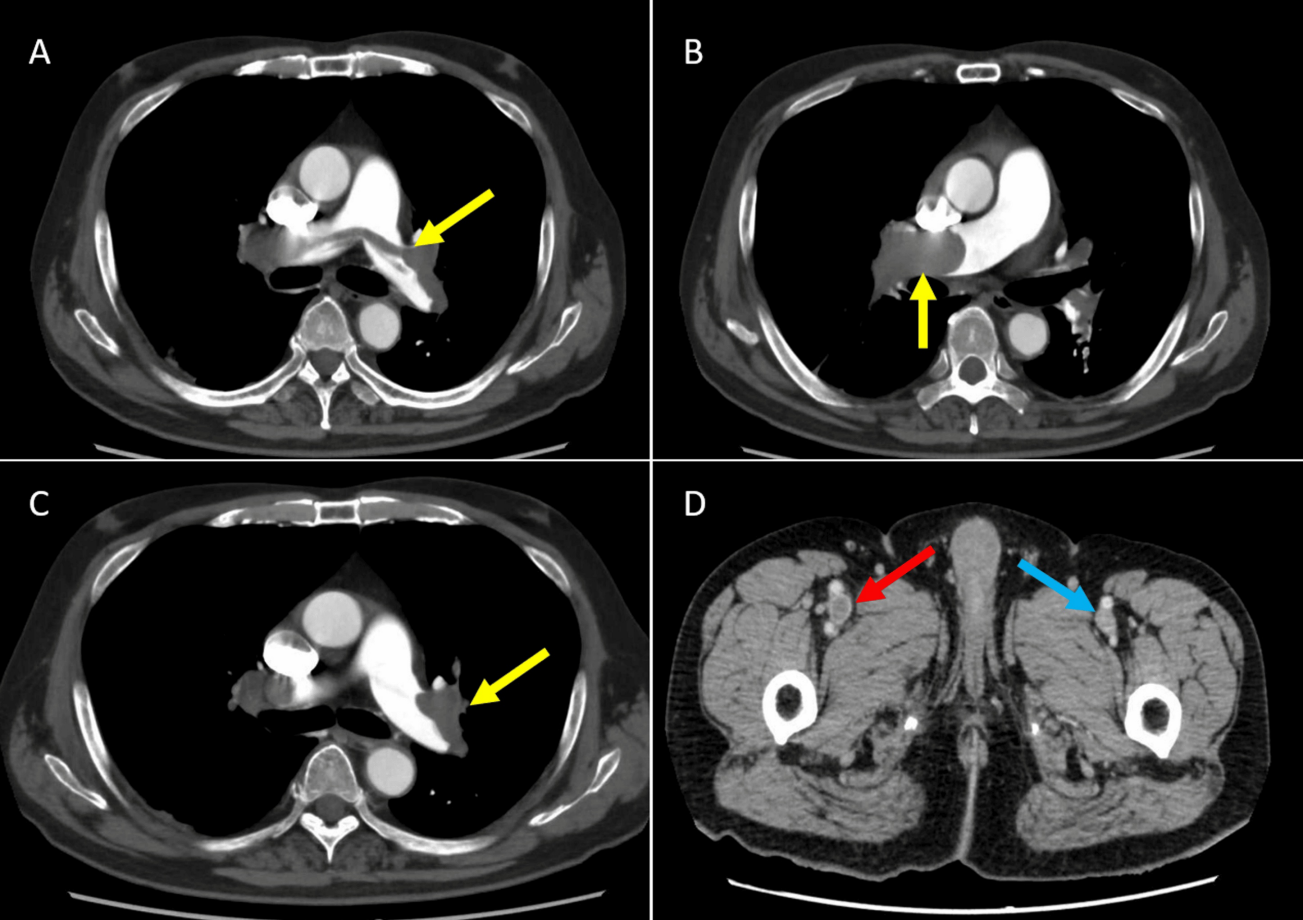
CT scan images at admission Contrast-enhanced CT images at presentation show evidence of acute pulmonary embolism and deep vein thrombosis. A, B, and C demonstrate filling defects within the pulmonary arteries (yellow arrows), consistent with bilateral thromboembolism. D shows a filling defect in the right femoral vein (red arrow), supporting a diagnosis of deep vein thrombosis, while no such defect was observed in the left femoral vein (blue arrow). These findings, alongside elevated D-dimer and right heart strain on echocardiography, confirmed systemic venous thromboembolism and prompted anticoagulation and thrombolytic therapy. CT, computed tomography
